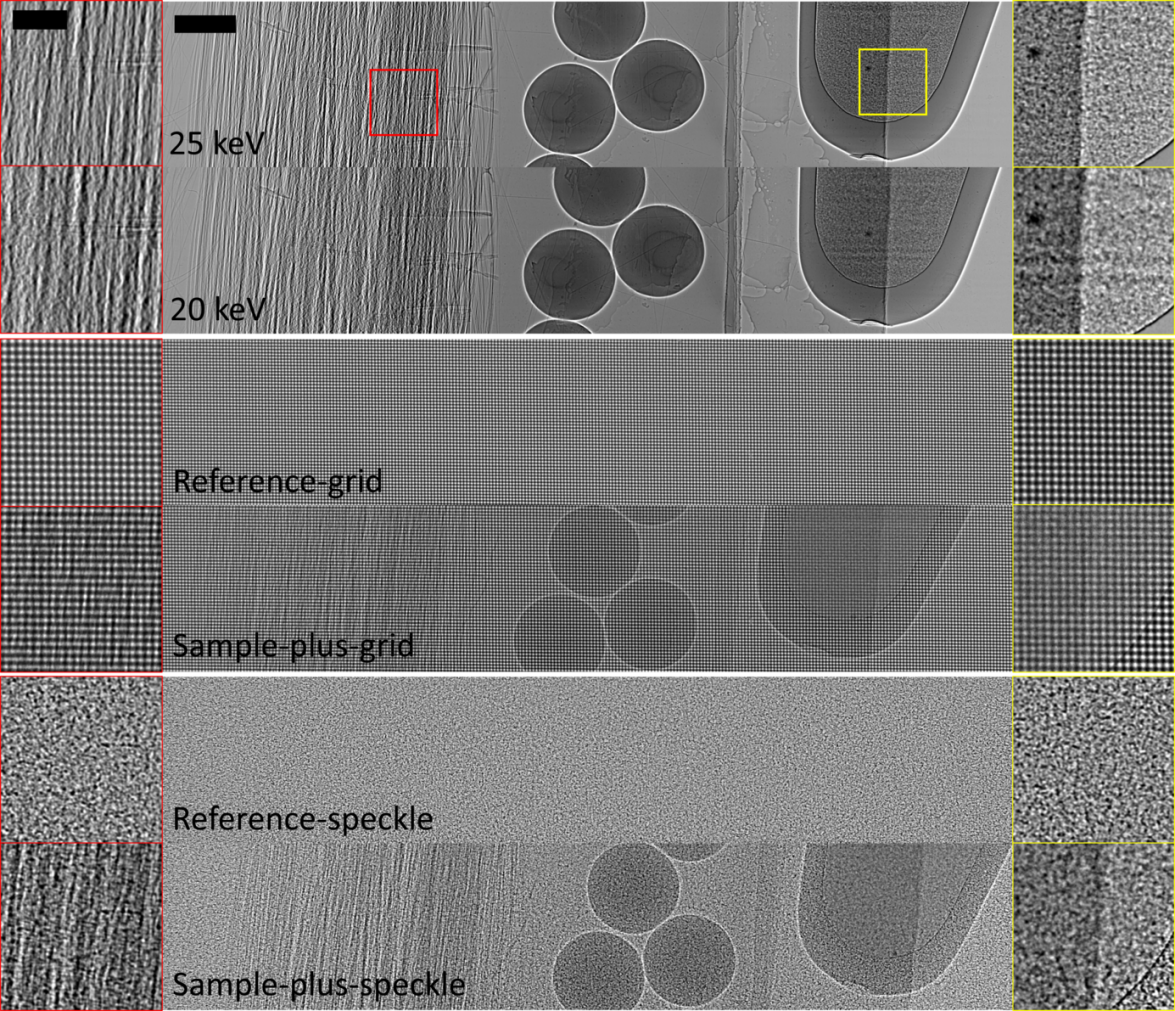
Images of the carbon-sphere sample, collected for the various dark-field approaches compared in this study. (Top) Dual-energy propagation-based approach. Dark-field contrast is generated by the difference in local blurring between two images collected at two sufficiently different X-ray energies (Ahlers *et al.*, 2024 ▸). Note that the horizontal features observed within the microsphere-filled tube at 20 keV originate from the flat-field correction. The illumination was not uniform, and the horizontal structures in it were blurred by the dark-field effects generated by the microspheres, preventing these variations from being fully divided out during the flat-field correction. This is analogous to the challenges of performing flat-field correction in the presence of strong phase effects (Homann *et al.*, 2015 ▸), but arises here due to dark-field effects. (Middle) The sample-induced dark-field effect in the single-grid approach blurs the 2D periodic reference-grid pattern (How & Morgan, 2022 ▸). (Bottom) In speckle-based imaging, dark-field contrast can be retrieved by tracking the speckle-blur between the reference-speckle and sample-plus-speckle images (Alloo *et al.*, 2023 ▸; Beltran *et al.*, 2023 ▸). The two black rectangles in the top left-hand images of this figure indicate the scale bars for the magnified and whole images (0.2 mm and 1 mm, respectively). Note that in the case of the multi-speckle approach, images are collected for multiple positions of the speckle generator, but these are not shown here.
